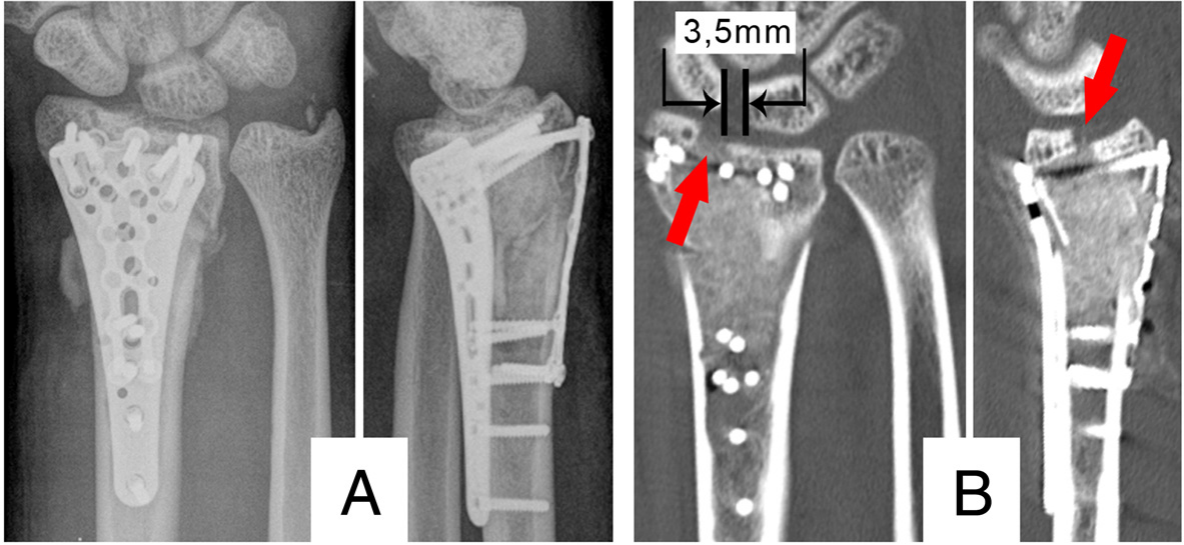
Postoperative computed tomography. A) Postoperative radiographs; B) postoperative computed tomography, the red arrows show a 2-3 mm intra articular gap and the scapholunate ligament dissociation of 3,5 mm is marked.
